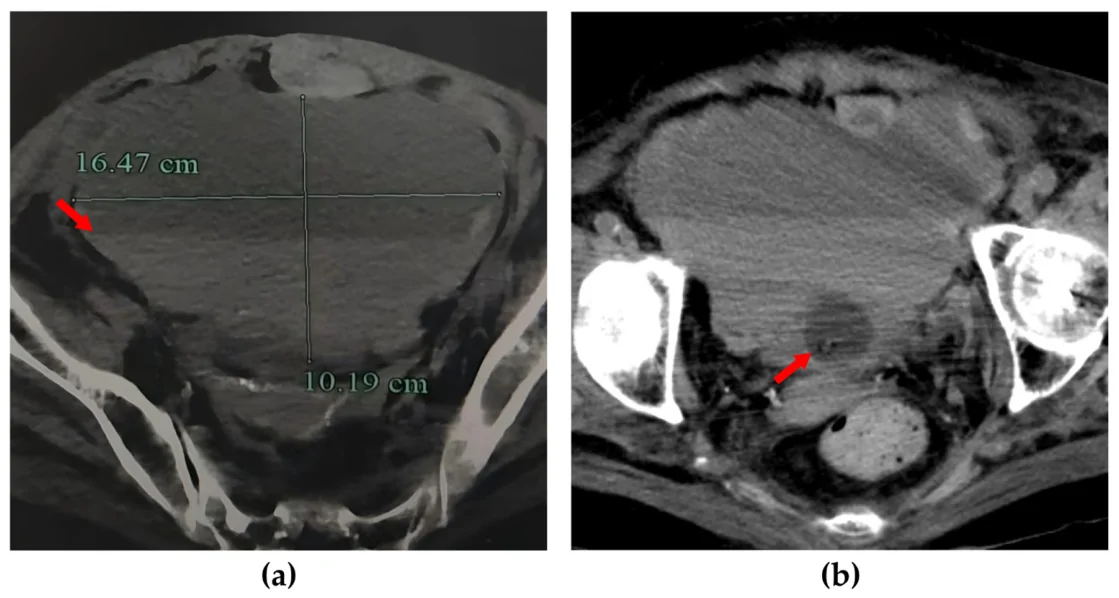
Non-contrast abdominal CT. (a) A giant, bladder-shaped rectus sheath hematoma with an internal fluid-fluid level (arrow). (b) The urinary catheter (arrow) remains outside the fluid-filled mass without entering its cavity.
